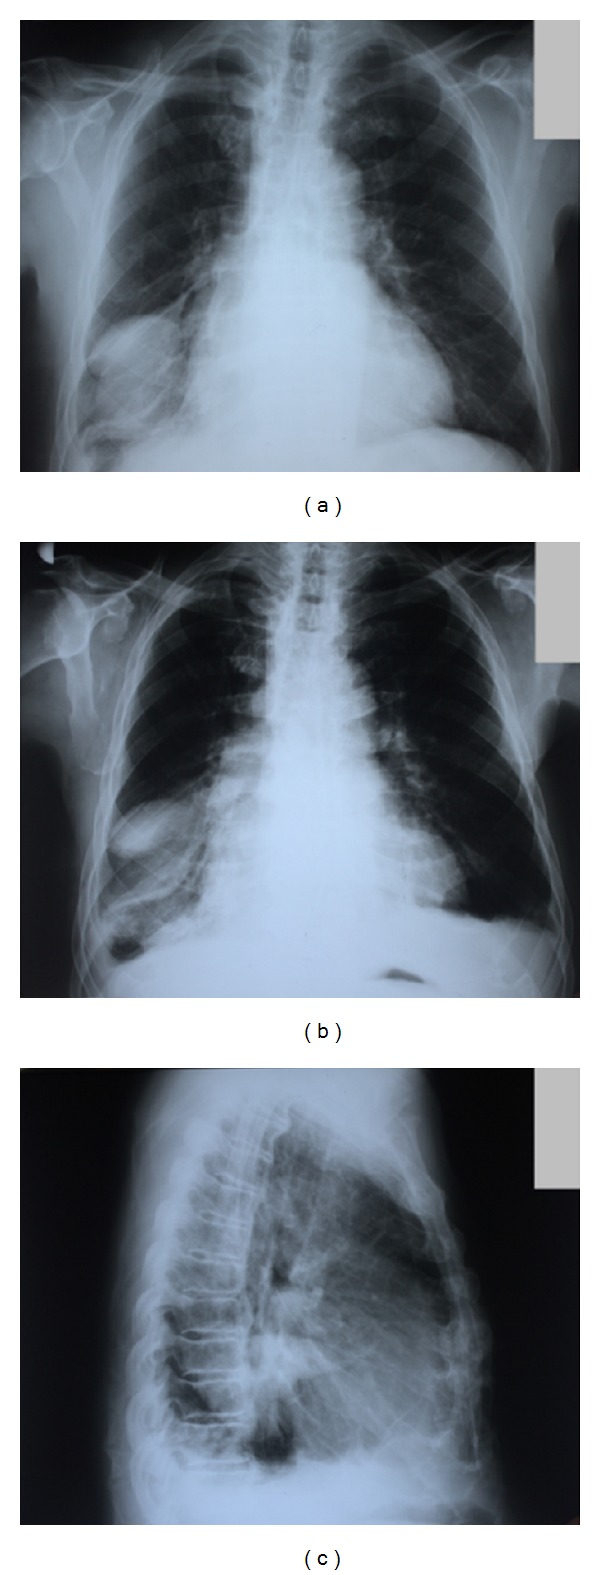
Archive chest-X-ray. (a) Chest-X-ray examinations undergone 4 years prior to admission showed similar tumor-like shadows in the same region of the right lobe. (b) Posteroanterior CXR undergone 1 year prior to admission demonstrating similar homogeneous shadows in the region of the right lower lung. (c) Lateral CXR undergone concurrently 1 year prior to admission demonstrating well demarcated opacity in the posterior aspect of the chest.
